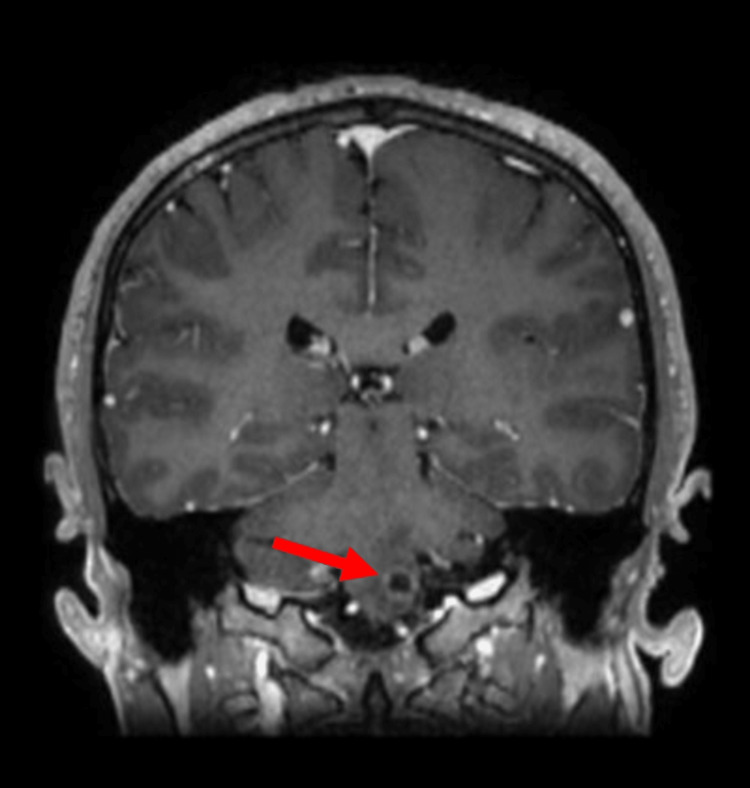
Coronal T1-weighted contrast-enhanced brain MRI demonstrating a hypointense ring-enhancing lesion at the left medulla oblongata (arrow).
